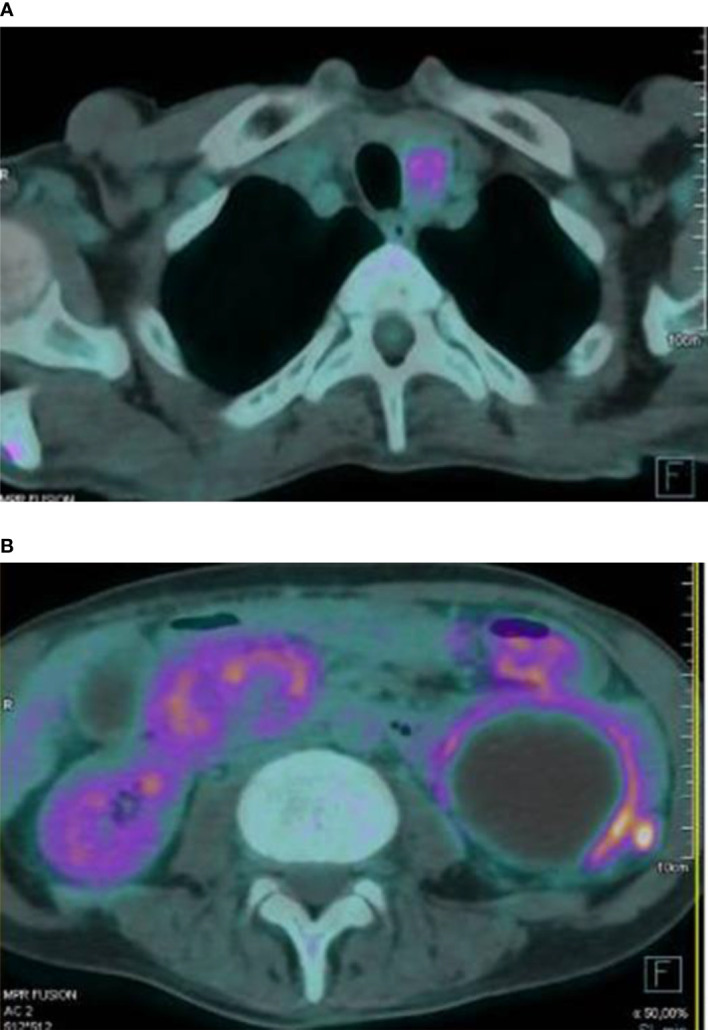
Intense glucose uptake in the anterior neck region was observed at 18-FDG PET/CT scan (A). The exam also documented a peripheral mild glucose uptake from several abdominal masses (B).
